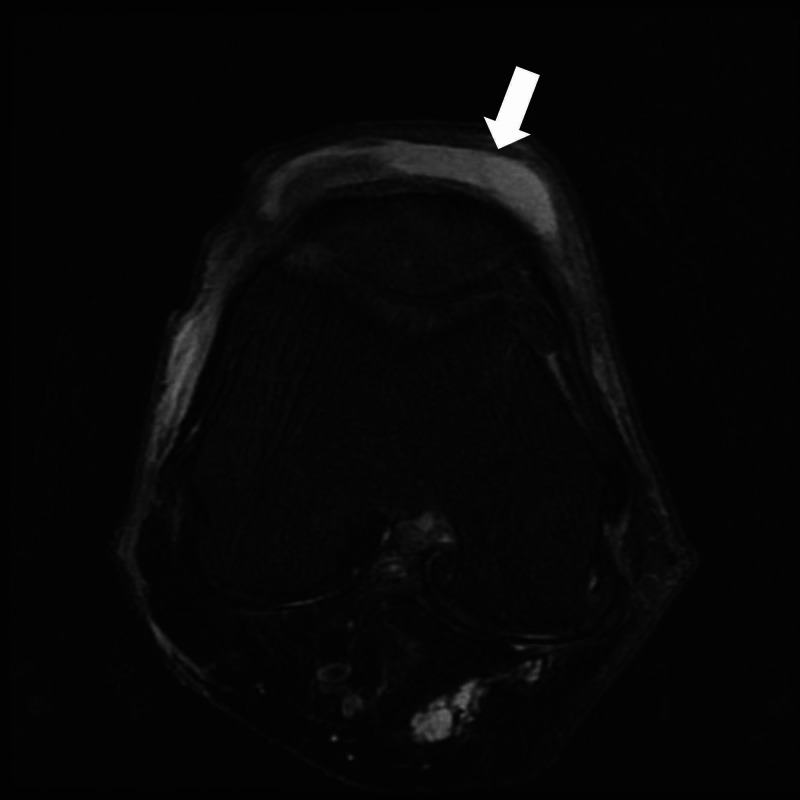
T2 fat suppression MRI scan (Axial view) showed hyperintensity area in the prepatellar bursa of right knee.
